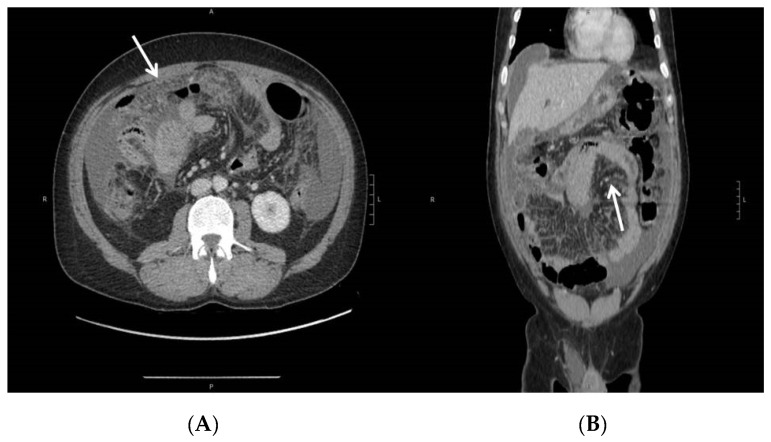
Abdominal CT—peritoneal effusion, diffuse suffusion of mesenteric adipose tissue, and thickening of the parietal peritoneum as shown in axial (A) and coronal (B) views. Diffuse parietal thickening (arrows) of the ileo-jejunal loops, with preserved parietal enhancement.
